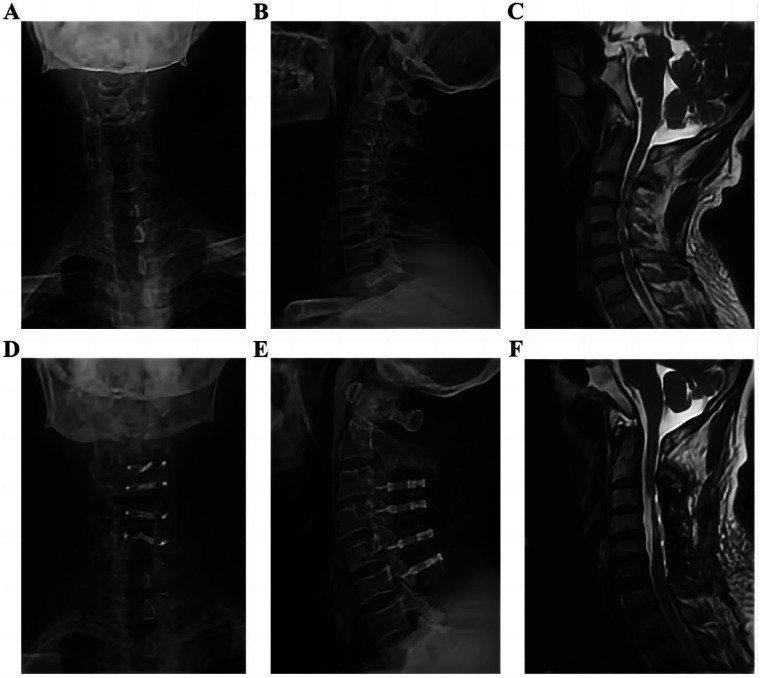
Patient 2, a 57-year-old male, came to the hospital with acute pain and weakness in both upper extremities after a trauma that occurred three hours before admission. He had a history of cervical spinal stenosis resulting in central canal syndrome at multiple levels. A posterior single-door laminoplasty was conducted, and radiographs [(A,B) films] demonstrated normal cervical lordosis without significant intervertebral space narrowing. Preoperative cervical MRI (C) revealed multiple instances of cervical spinal stenosis accompanied by high signal changes in the C3–5 spinal cord region. Postoperatively, radiographs [(D,E) films] showed satisfactory positioning of internal fixation devices, and postoperative cervical MRI [(F) film] revealed noticeable posterior displacement of the spinal cord without significant compression.
